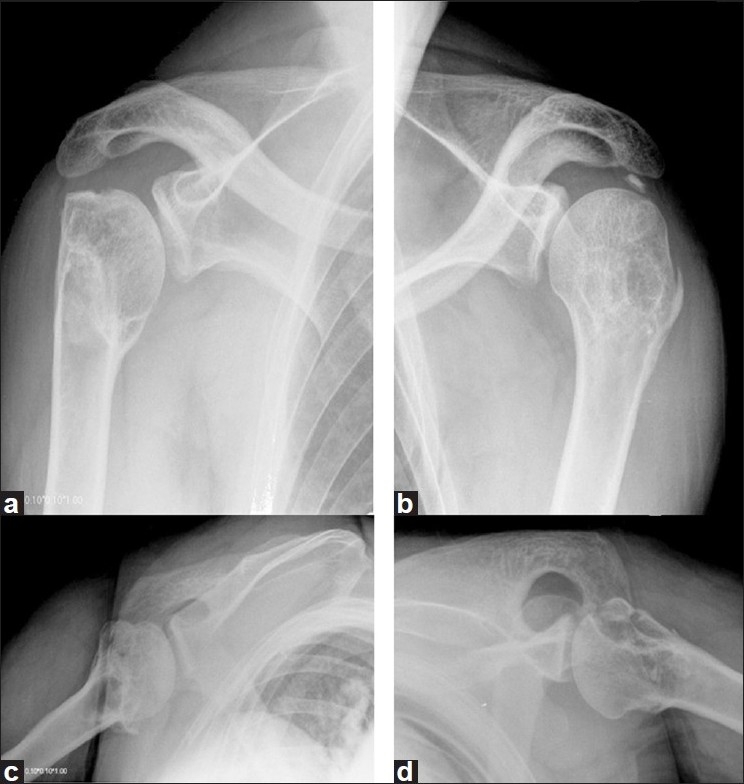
Radiographs of both shoulders [right- (a,c); left- (b,d)] 27 months after trauma, showing consolidation of the fractures without signs of avascular necrosis
